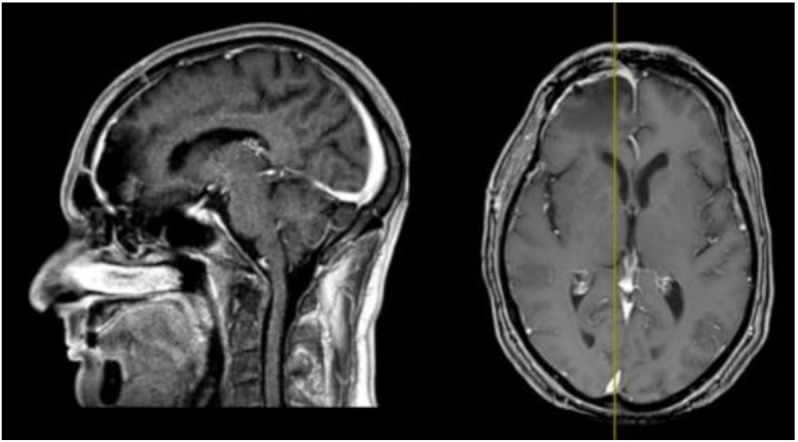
Post-operative brain MRI T1-weighted with contrast images showing the complete removal of the anterior cranial fossa meningioma.
